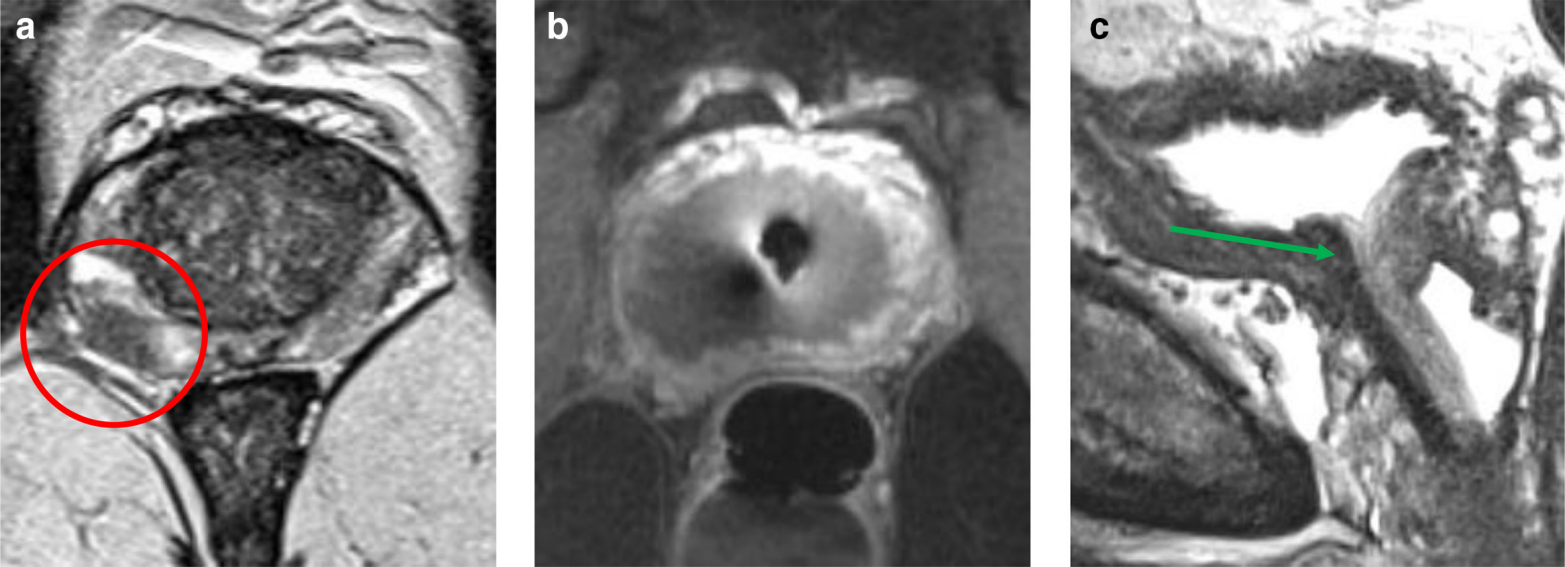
Transurethral ultrasound ablation MRI of a 69-year-old male with an initial PSA of 6.0 ng ml^−1^. Patient underwent MRI-guided whole gland TULSA as treatment for a Gleason score 3+4=7 lesion at the right peripheral zone (PI-RADS 5). (a) Axial *T*
